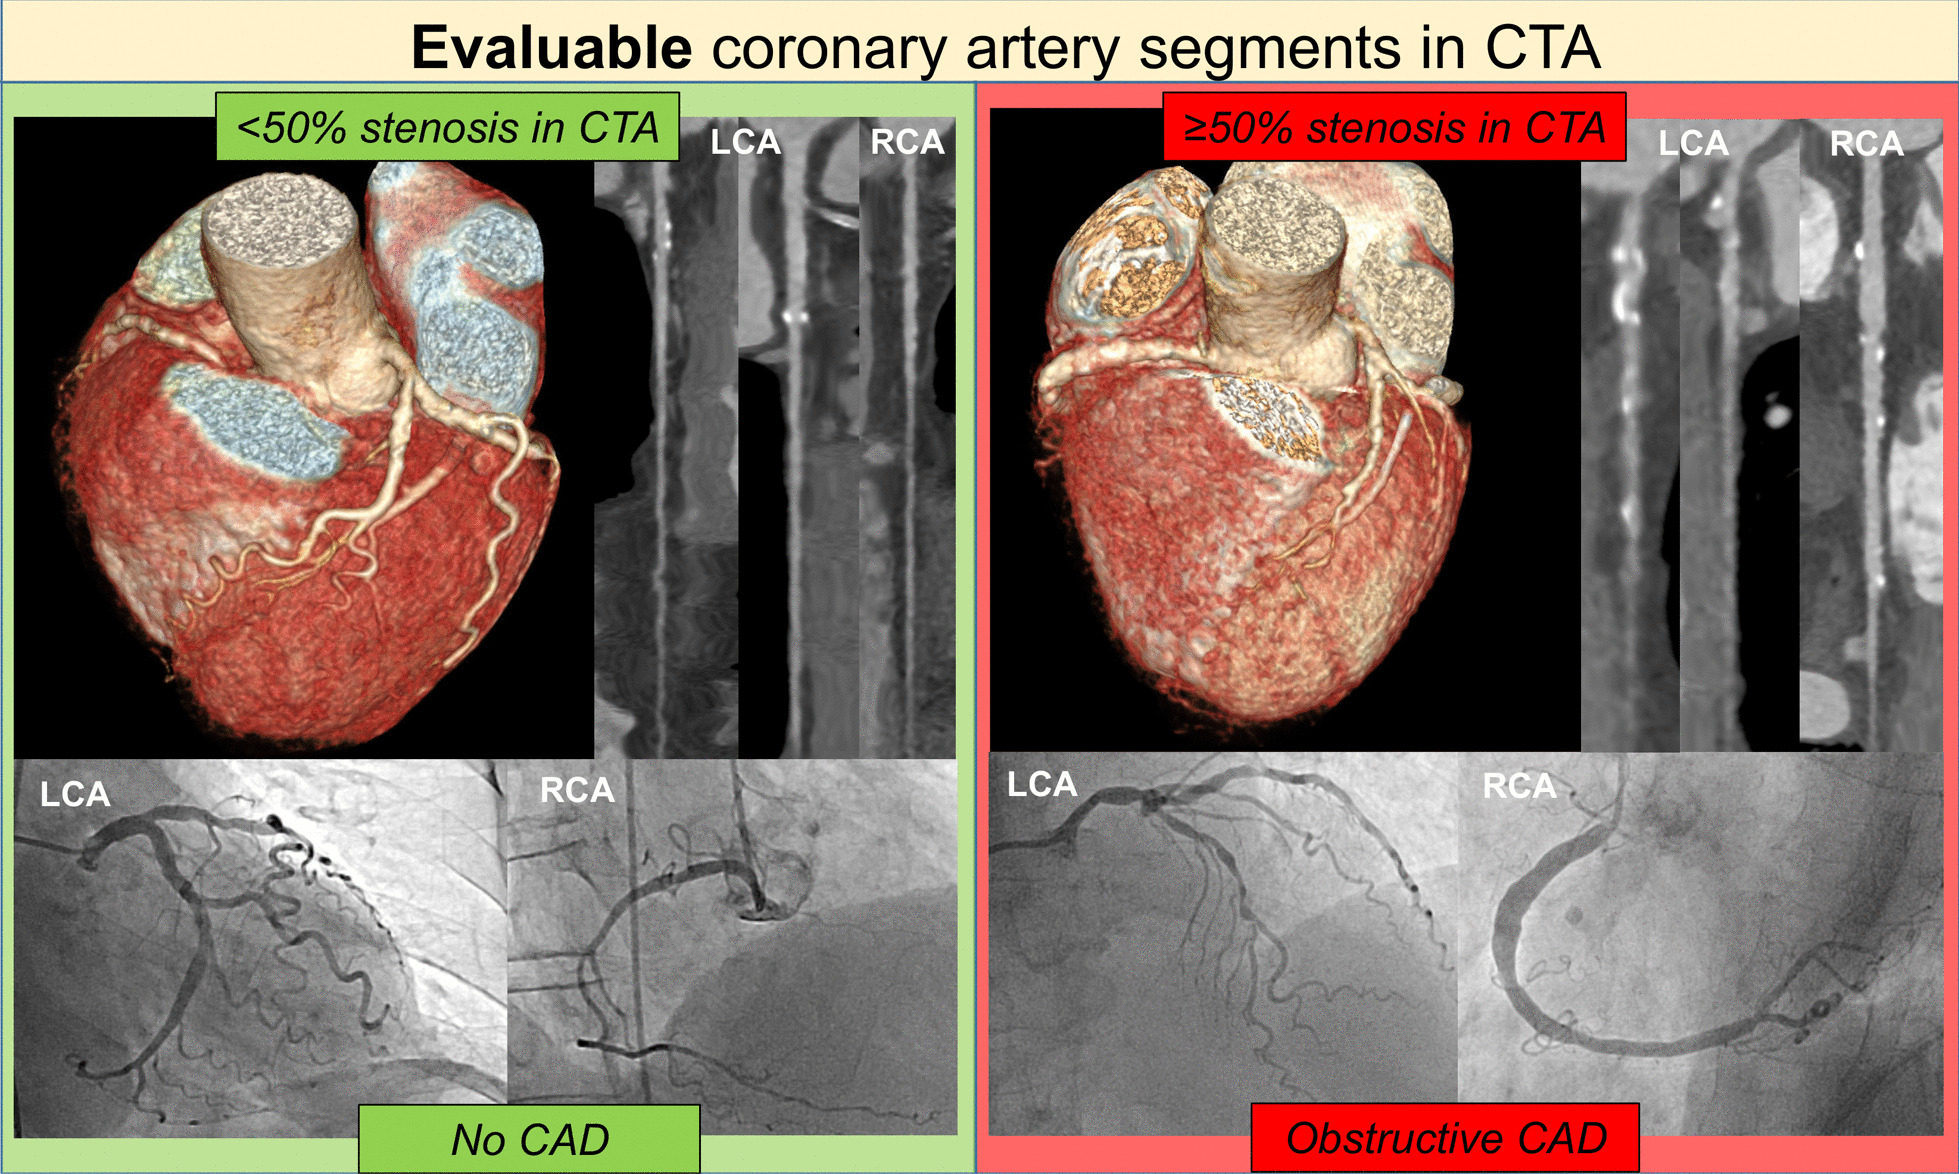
Examples of patients undergoing pre-TAVI CT with “evaluable” coronary artery segments. On the left side a patient is presented where in the pre-TAVI CTA all coronary segments were evaluable. There was no obstructive CAD present in CTA analysis, confirmed by invasive QCA. On the right hand side, a patient is presented, where CTA showed obstructive CAD, confirmed by invasive QCA in the distal left main stem and in the LAD. *CAD* coronary artery disease, *CTA* computed tomography angiography, *RCA* right coronary artery, *LCA* left coronary artery
